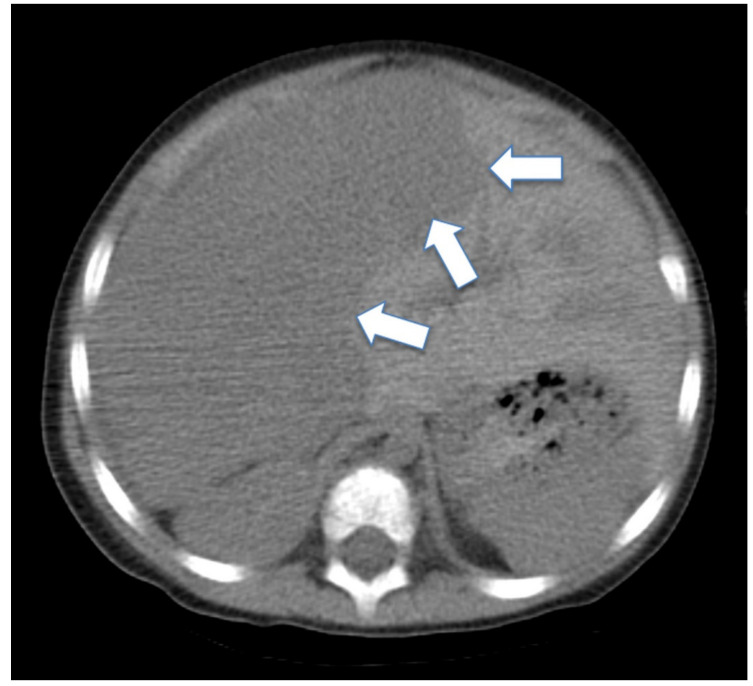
CT scan of the abdomen without contrast at the diagnosis of hepatoblastoma. It demonstrates the right liver mass measuring 10 × 10 × 15 cm. The white arrows show the line between normal and neoplastic liver tissue. The arrows are within the normal liver tissue.
